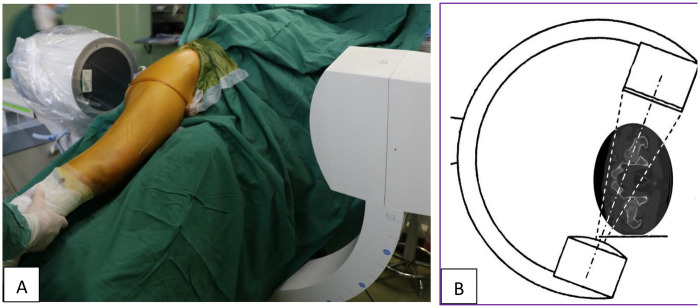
Intraoperative fluoroscopy in the lateral decubitus position: for the anteroposterior (AP) view, position the C-arm x-ray tube perpendicular to the patient's hip (A); for the lateral view, tilt the C-arm 20° cephalad to obtain a coaxial image of the femoral neck and shaft (B).
